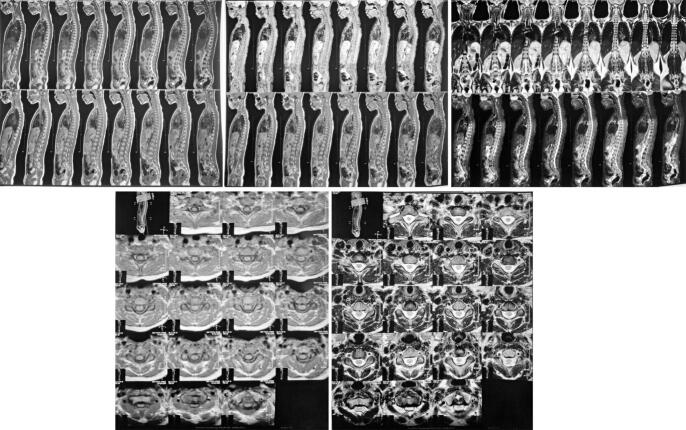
MRI Cervical-Thoracal-Lumbal-Sacral on axial, coronal, and sagittal view confirmed retrolisthesis of odontoid and severe canal stenosis along with spinal cord edema on level VC2.
